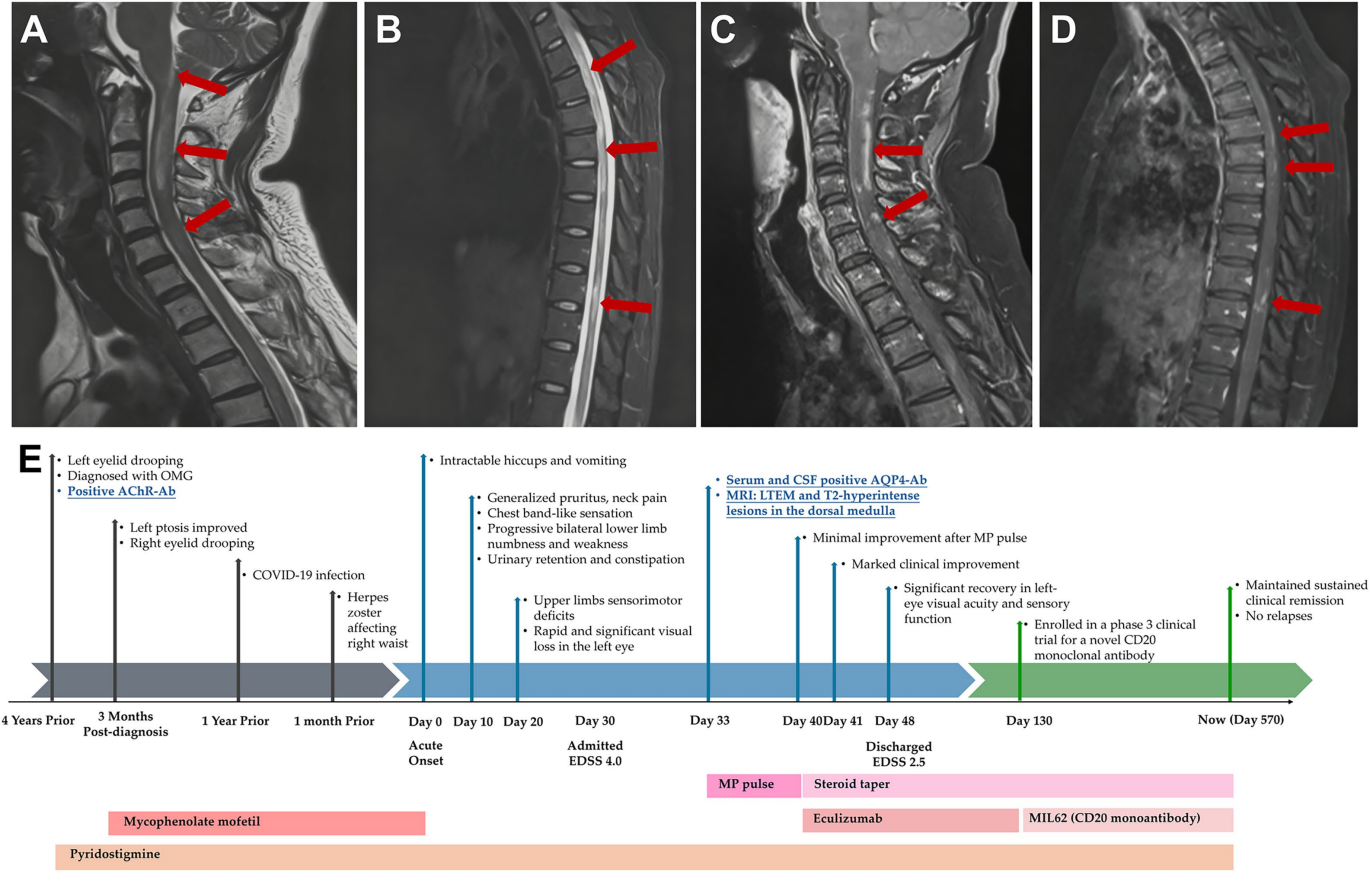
Magnetic resonance imaging and clinical timeline of the patient. (A–D) MRI of the patient at time of admission: sagittal view of the cervical and thoracic spine revealed multiple T2-weighted hypersignal lesions in the posterior bulbar (area postrema), cervical (A) and thoracic medulla (B), with some lesions showing contrast enhancement in contrast-enhanced T1-weighted images (C,D; arrows). (E) Detailed graphical timeline of the patient’s symptoms. IVMP, intravenous methylprednisolone; EDSS, Expanded Disability Status Scale.
